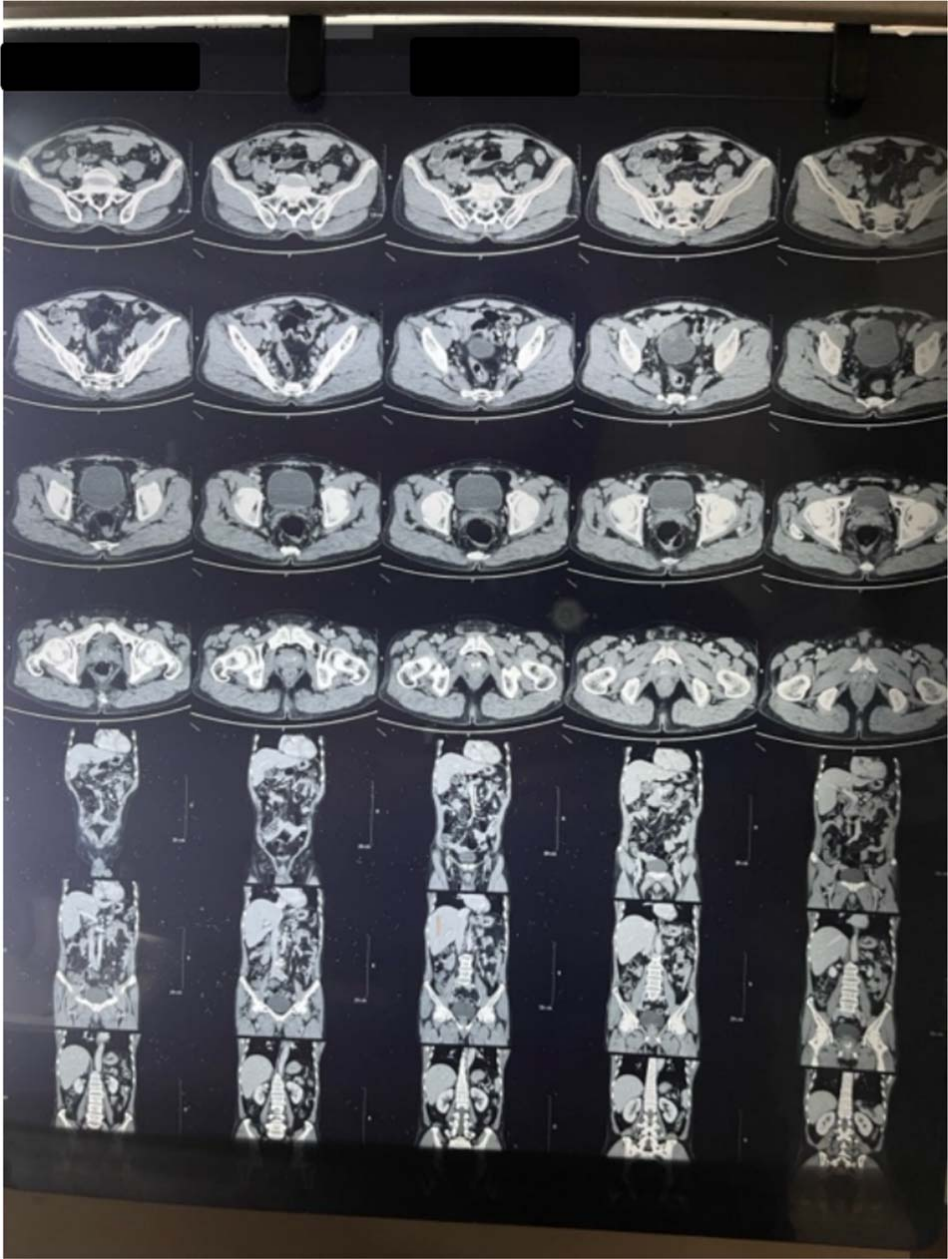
CT coronal cut abdomen and pelvis shows thickened wall.
